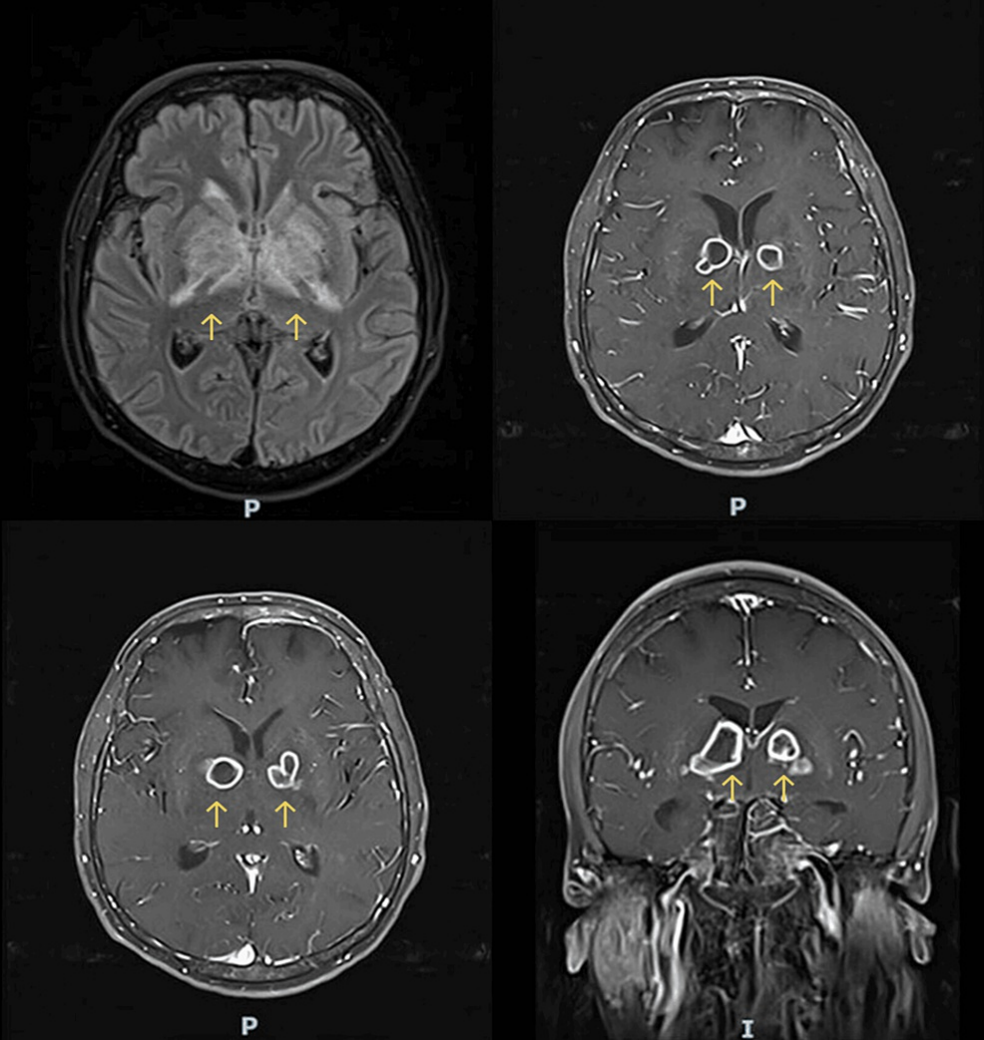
Initial brain MRI showing ring-enhancing lesion affecting both basal ganglia with surrounding edema MRI: magnetic resonance imaging
